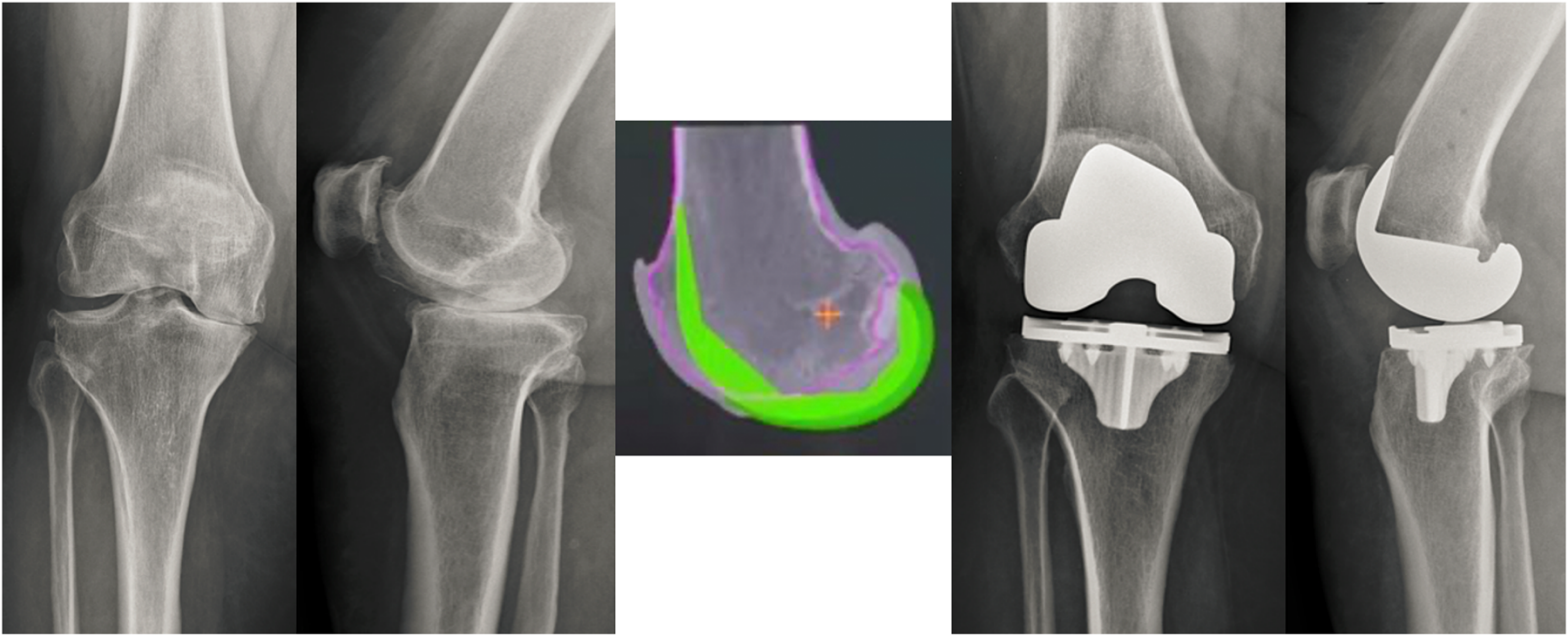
A 70‐year‐old woman with anterior compartment understuffing by trochlear understuffing at 30° and 70° of flexion. Preoperative and postoperative radiographs (AP and lateral views) and robotic planning in the sagittal plane.
